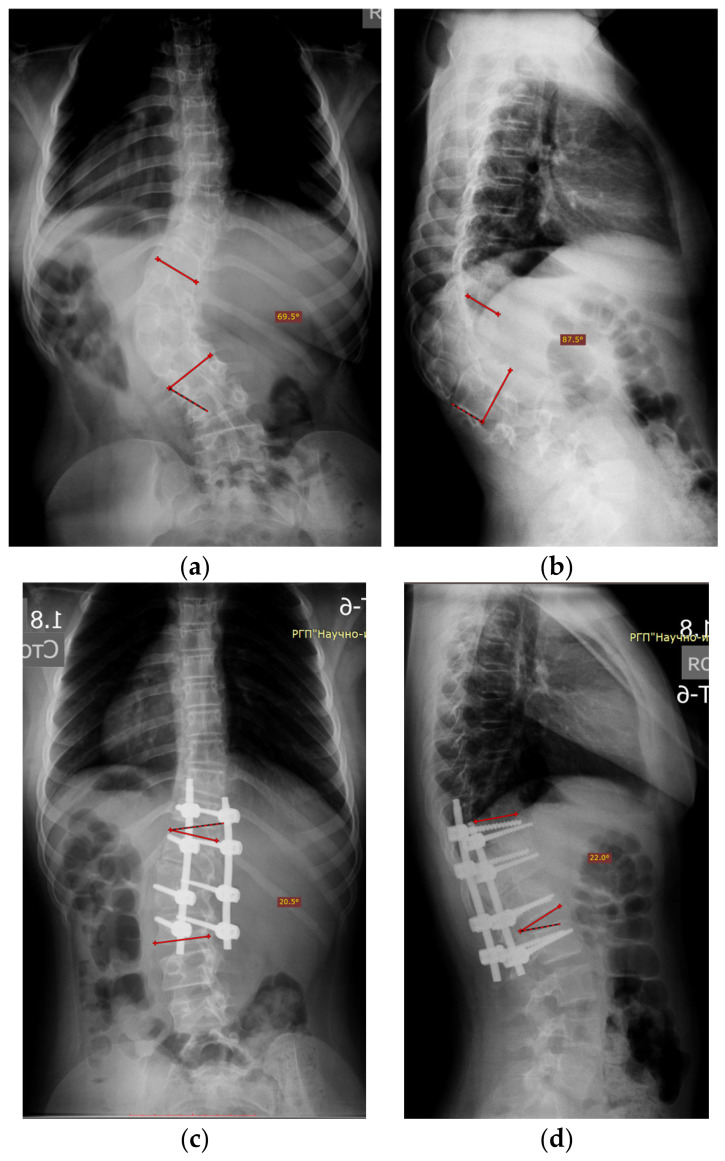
Representative case of congenital spinal deformity correction: (a) Preoperative anteroposterior radiograph demonstrating congenital spinal deformity associated with a structural vertebral anomaly; (b) preoperative lateral radiograph; (c) postoperative anteroposterior radiograph after posterior instrumented correction and fusion showing improved coronal alignment; (d) postoperative lateral radiograph showing improved sagittal alignment after correction.
